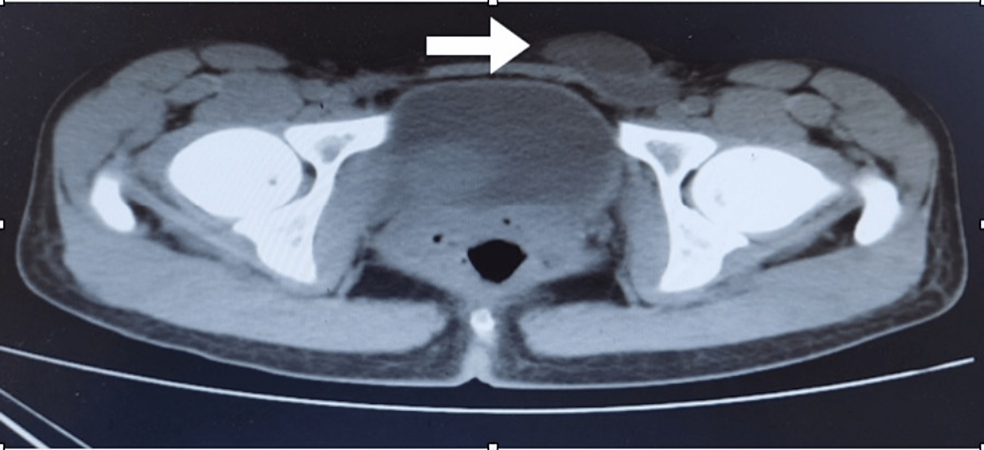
Abdominopelvic CT scan without contrast shows a lobulated hypodense lesion in the left inguinal area with connection to the deep inguinal region (arrow).
